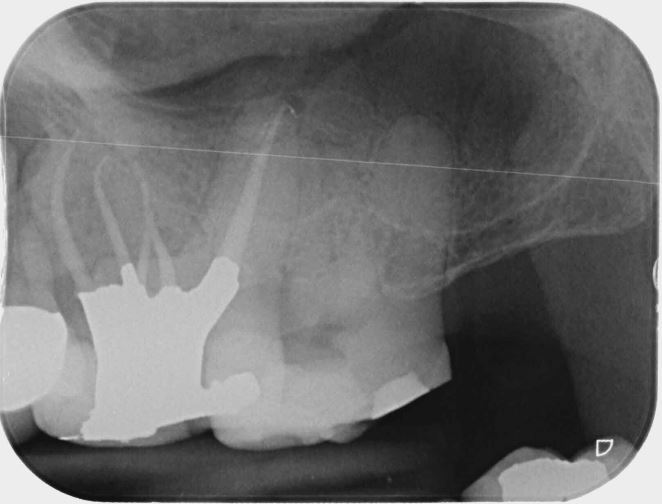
Postoperative periapical radiograph of root treated upper left first molar showing a ‘skinnier' style of preparation than previously produced
